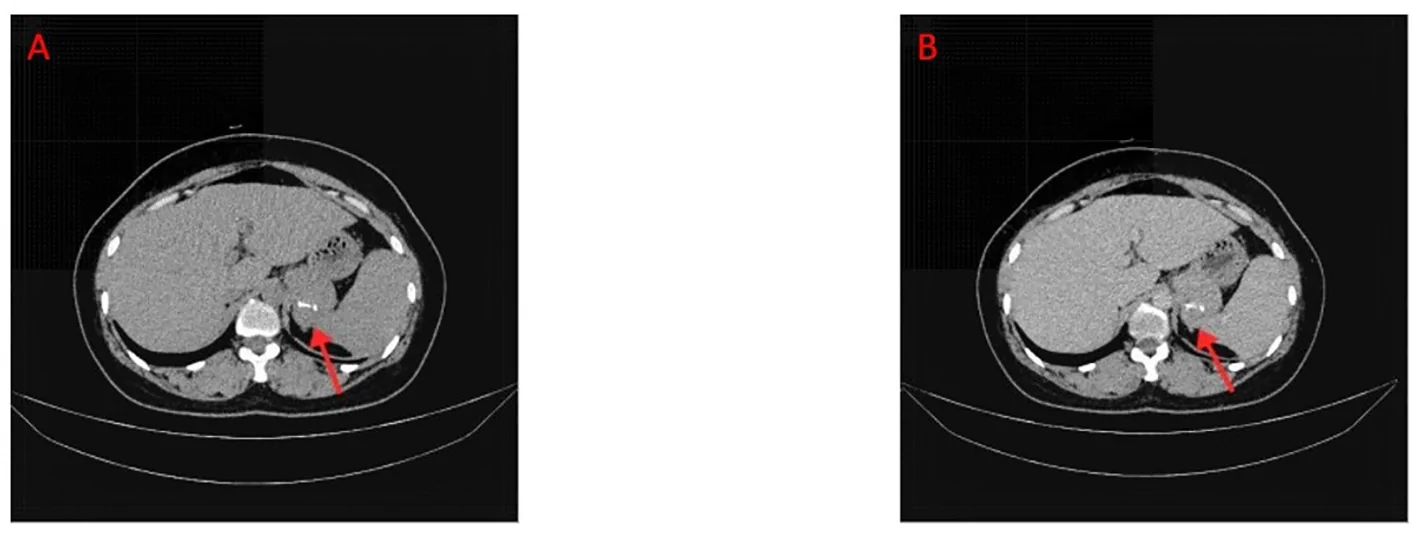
Imaging findings of the left adrenal lesion. (A) Plain CT scan: A well-defined heterogeneous mass measuring approximately 53 mm × 42 mm is observed in the left adrenal region, with internal linear and punctate calcifications. (B) Contrast-enhanced CT scan: The left adrenal mass presents obvious enhancement. The native left adrenal gland is compressed and displaced downward (arrow).
